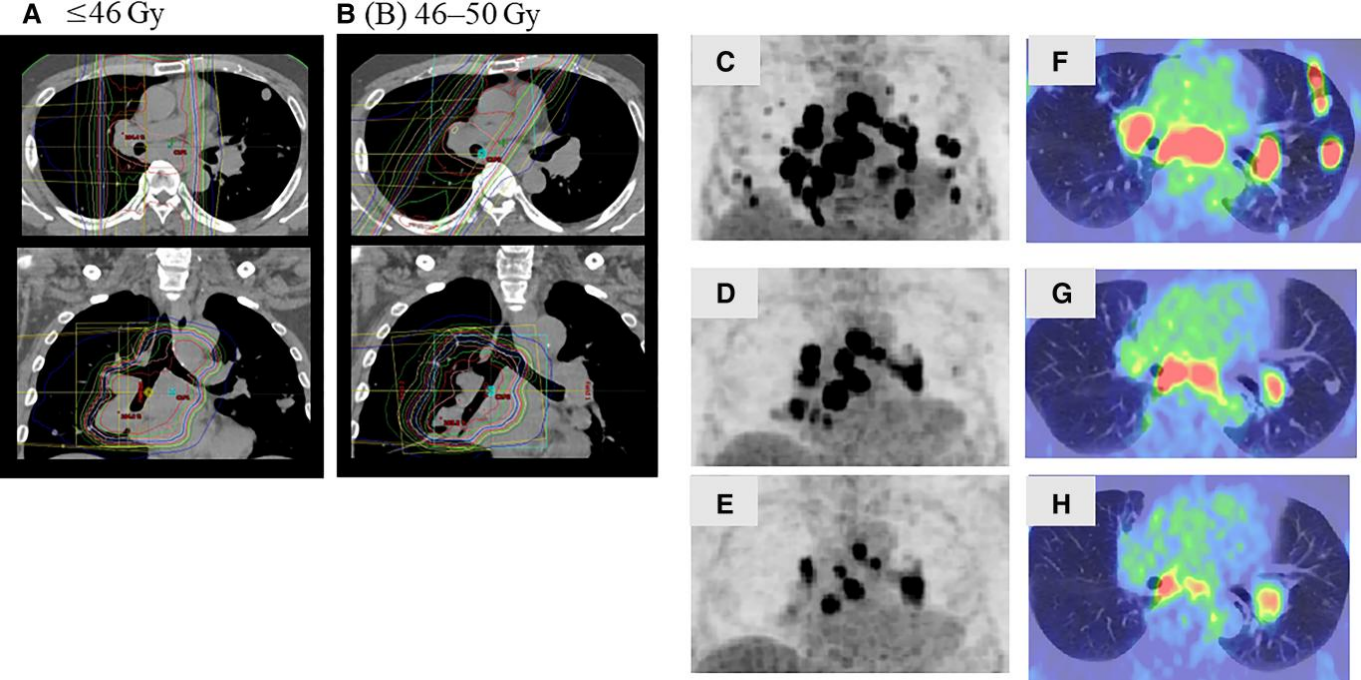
Dosimetry of 3D conformal radiotherapy (A, B) and ^18^F-FDG-PET (C-E) and ^18^F-FDG-PET/CT (F-H) findings 2 months before EBRT (C, F), 6 months (D, G), and 10 months (E, H) after EBRT. The lesions were treated using a 3D conformal radiotherapy total dose of 50 Gy administered in 25 fractions (dosimetry of ≤46 Gy and 46-50 Gy is shown in A and B, respectively). ^18^F-FDG-PET/CT showed shrinkage of the right mediastinal lymph nodes within the irradiated area with a decreasing SUV. Additionally, the left hilar lymph nodes and multiple lung metastases outside the irradiated area also decreased in size or disappeared. EBRT, external beam radiation therapy; 3D, 3-dimensional; FDG-PET, ^18^F-fluorodeoxyglucose-positron emission tomography; CT, computed tomography; SUV, standardized uptake value.
